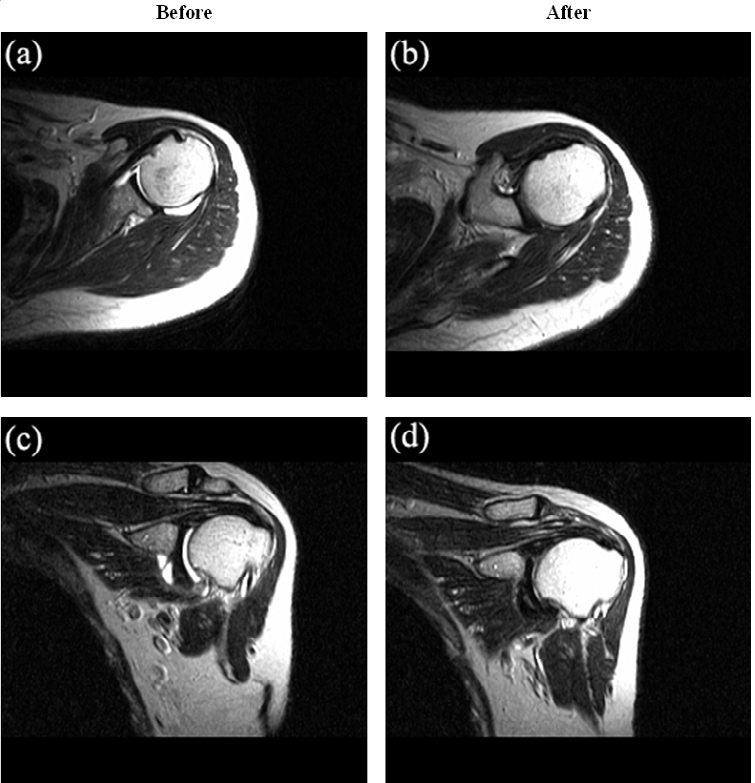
Magnetic resonance images (MRI) of a patient before and after interventional microadhesiolysis. The joint effusion decreased after the patient underwent interventional microadhesiolysis. (a) Axial and (c) coronal views of the shoulder MRI taken before intervention. (b) Axial and (d) coronal views of shoulder MRI taken after intervention.
